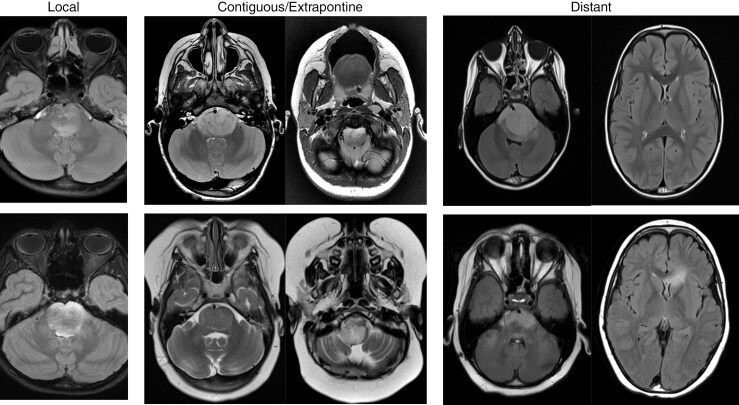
Representative axial MRI FLAIR or T2 sequences from 3 CED patients at 2 time points (t1 or t2 vs T3) demonstrating different anatomic/radiographic subtypes of progression. (Left) Local progression within the pons. (Middle) Improved local/pontine disease with new contiguous/extrapontine progression within the medulla. (Right) Improved local/pontine disease with distant progression in the corpus callosum. CED, convection-enhanced delivery.
